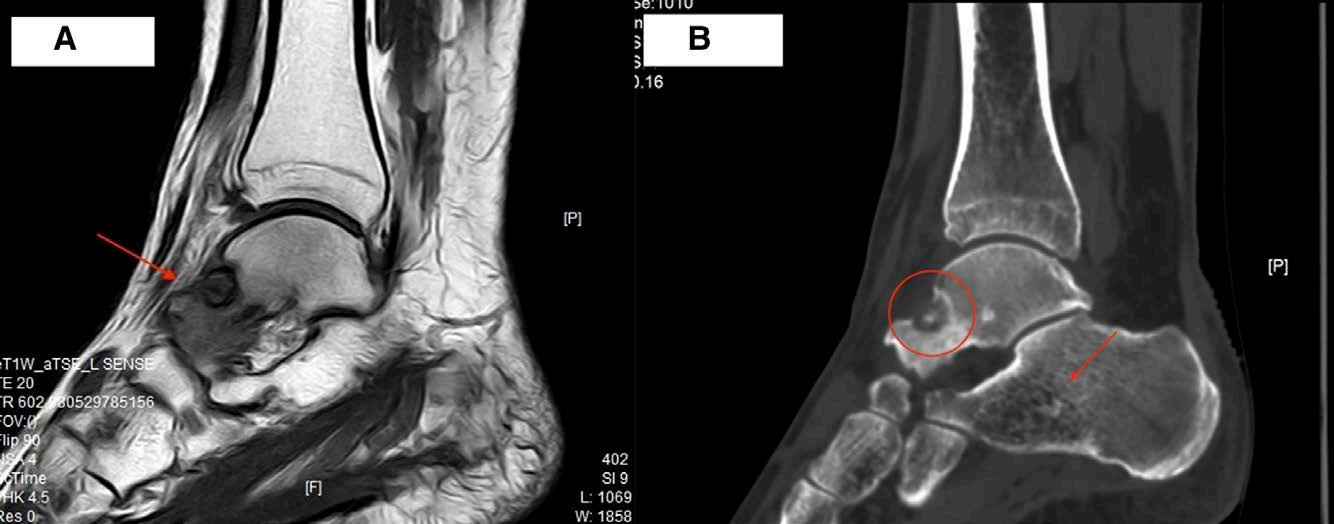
(A) MRI demonstrated localized bone marrow edema of the left talus and specific bone defects at the anterior superior border of the talus. (B) CT demonstrated an OO in the left talus, along with the formation of a calcaneal cyst and associated soft tissue swelling with effusion. CT = computed tomography, MRI = magnetic resonance imaging, OO = osteoid osteoma.
